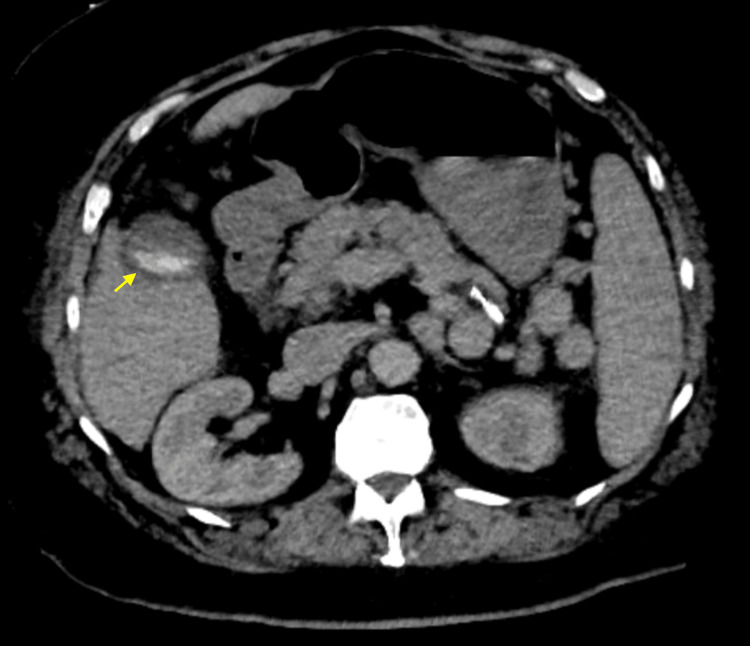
Axial contrast-enhanced CT abdomen Gallstones are present, along with some thickening and edema of the gallbladder wall. There is no biliary duct dilation or choledocholithiasis.
